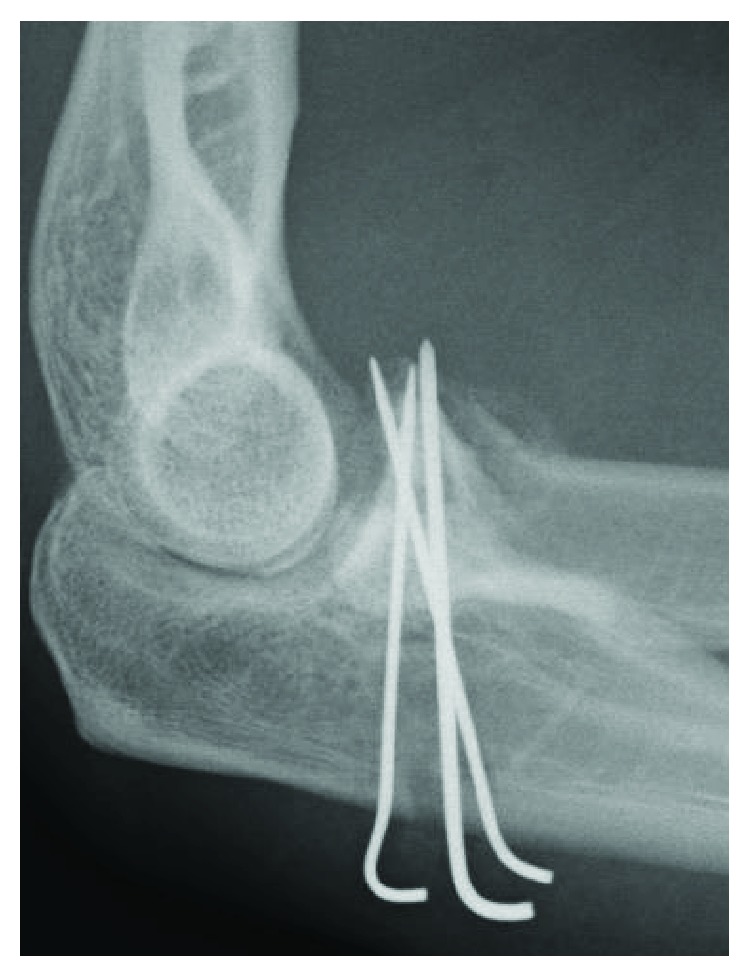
Case 3: postoperative radiograph shows fixation by three K-wires.
